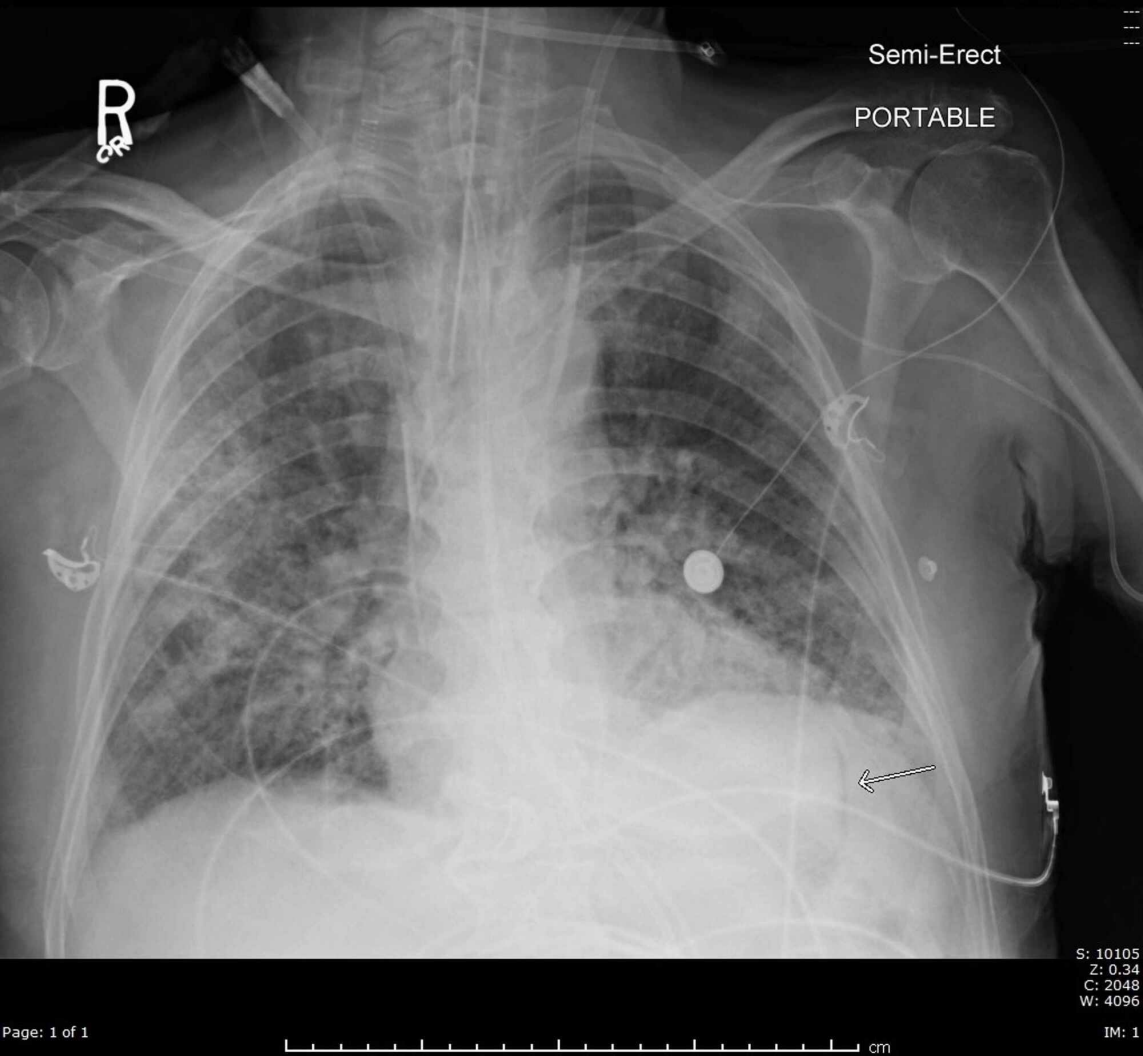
Chest X-ray showing pneumopericardium (arrow).
